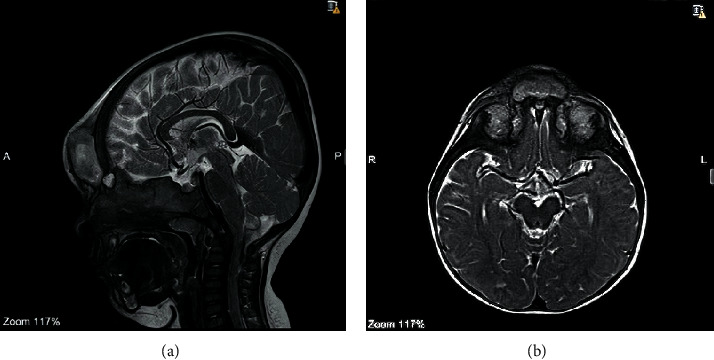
The patient's brain MRI in the T2-weighted sequence. The intracranial mass connected to the sinus tract and opening into the forehead abscess are shown in a (a) sagittal plane and (b) transverse plane.
